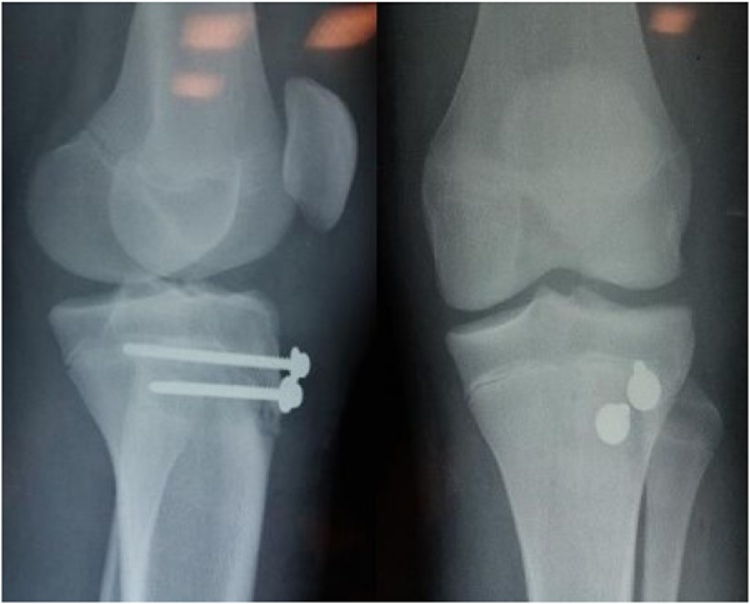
Post-operative X-rays showing a good reduction by two screws.
